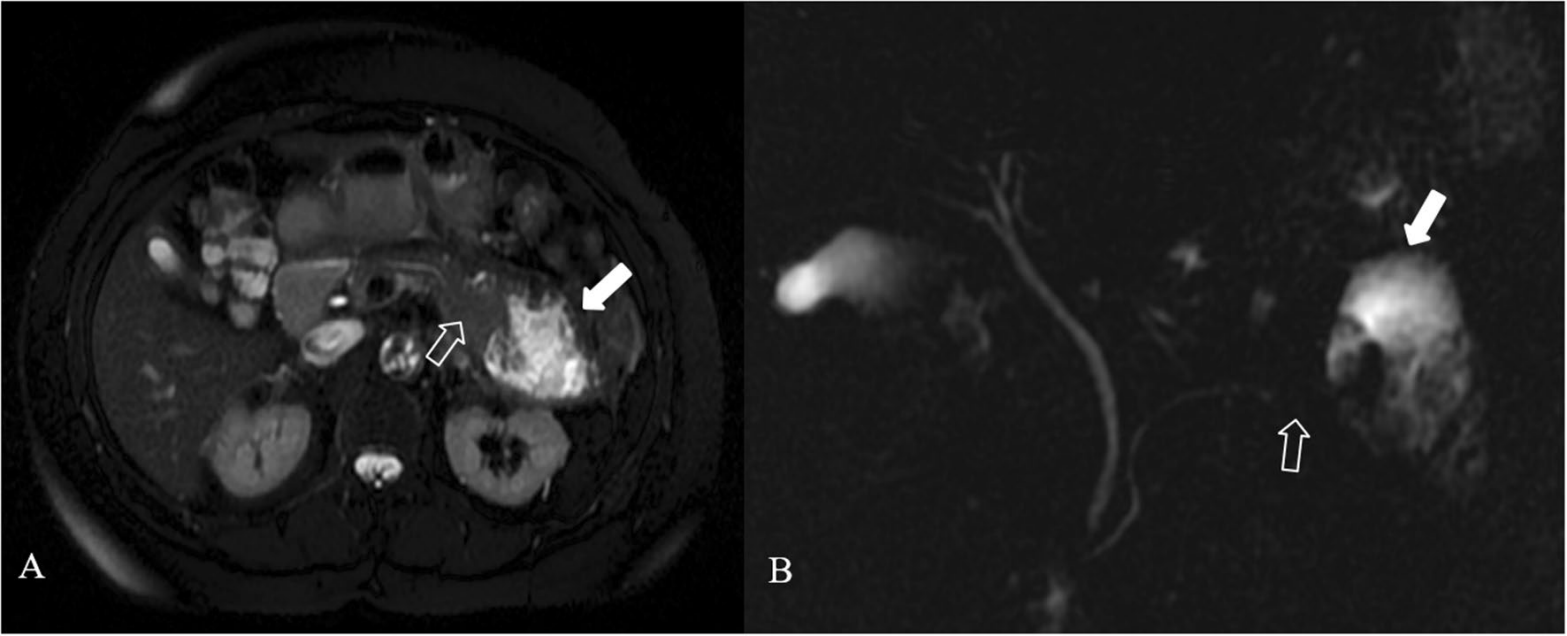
ACNs in a 72 years-old woman. T2 weighted MR image (a) and MRCP (b) 2 weeks after the onset show a heterogeneous collection in the pancreatic tail (white arrow), where there is a focal interruption of the Wirsung duct (empty arrow)
